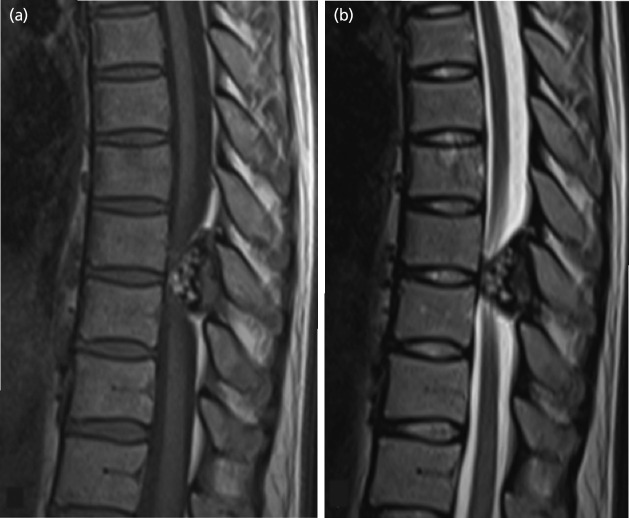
Case 9. MR images demonstrating a mass protruding into the spinal canal on the abdominal side of the lamina of Th9/10 level. (a) sagittal T1-weighted image, (b) sagittal T2-weighted image.
